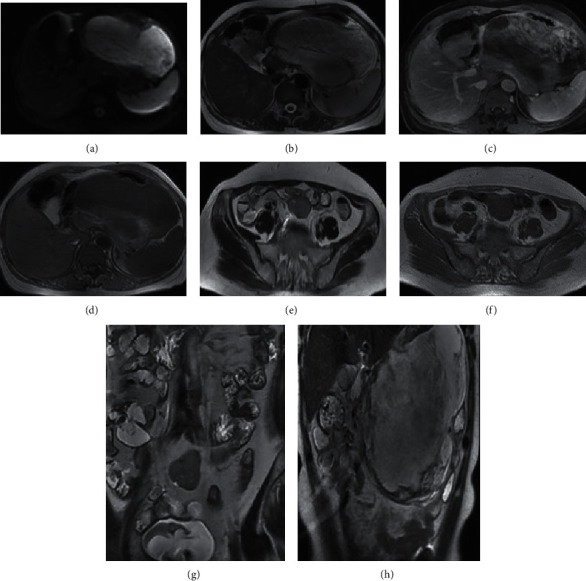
MRI image of patients with ileus. (a–d) Coronal MRI images; (e, f) transverse MRI images; and (g, h) aberrational MRI images.
